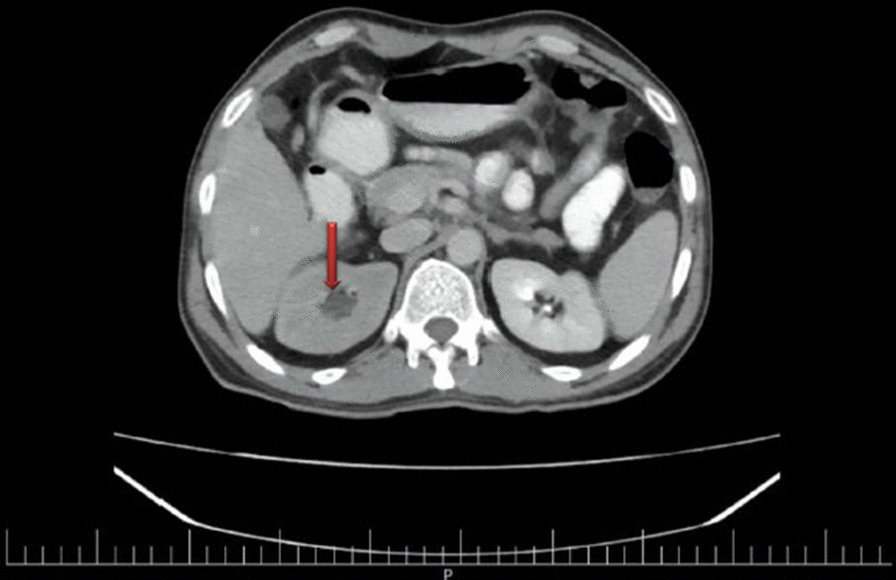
Abdominopelvic CT scan with contrast shows onset of hydronephrosis in the right kidney
